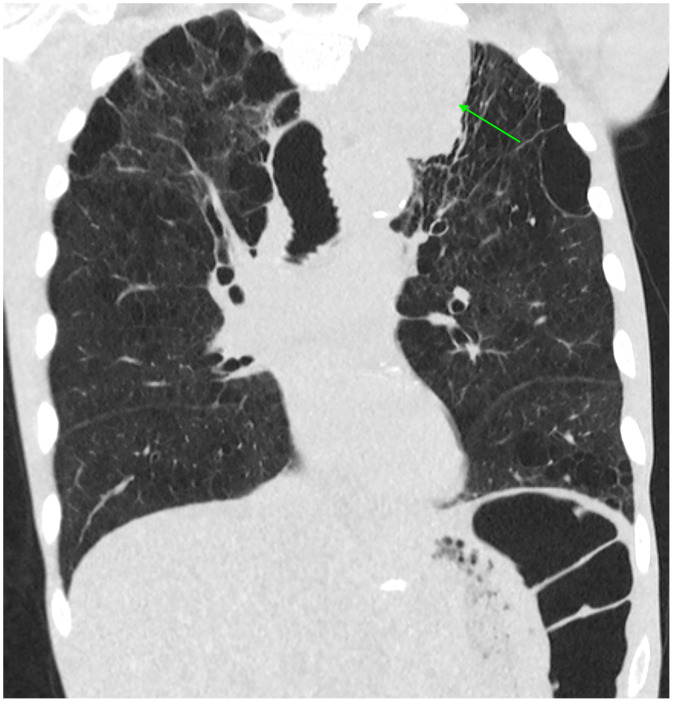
Computed tomography of the chest without contrast in coronal reformat demonstrates a 6.8 cm left apical lung mass (green arrow) representing the patient’s primary site of malignancy.
